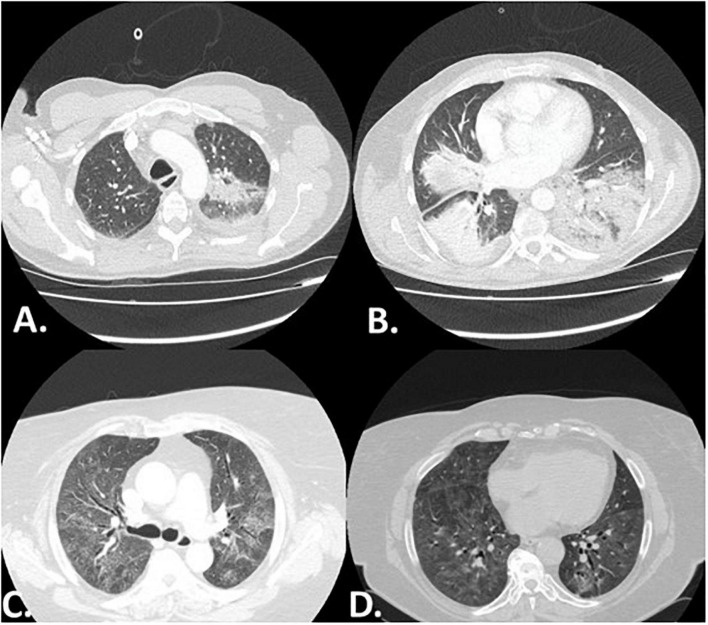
Representative high resolution computed tomography (HRCT) images of two patients with myositis associated-ILD. Panel (A,B) depict a patient with RP-ILD presenting with the radiographic pattern of organizing pneumonia. Panel (C,D) present a case of myositis in which non-specific interstitial pneumonia (NSIP) was observed 10 years following diagnosis.
